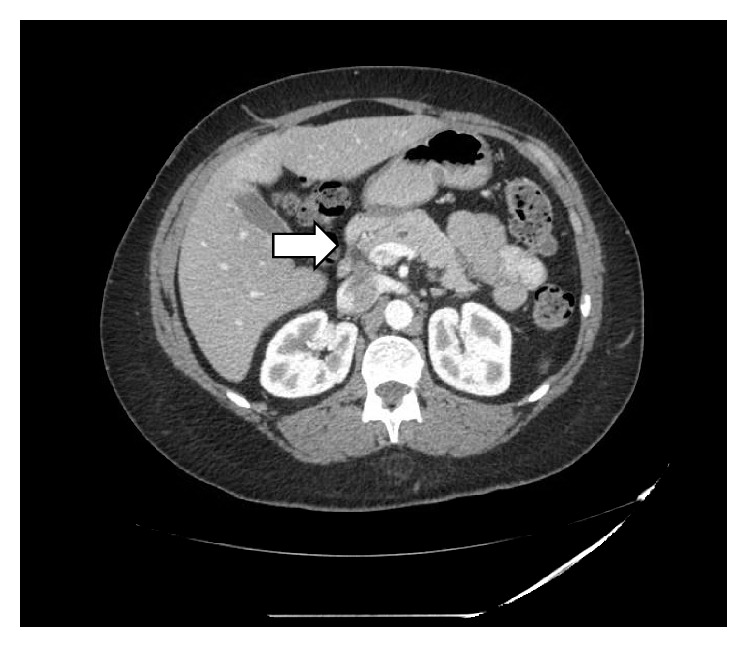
CT scan of the abdomen demonstrate a mass, arising from the uncinate process of the pancreas.
